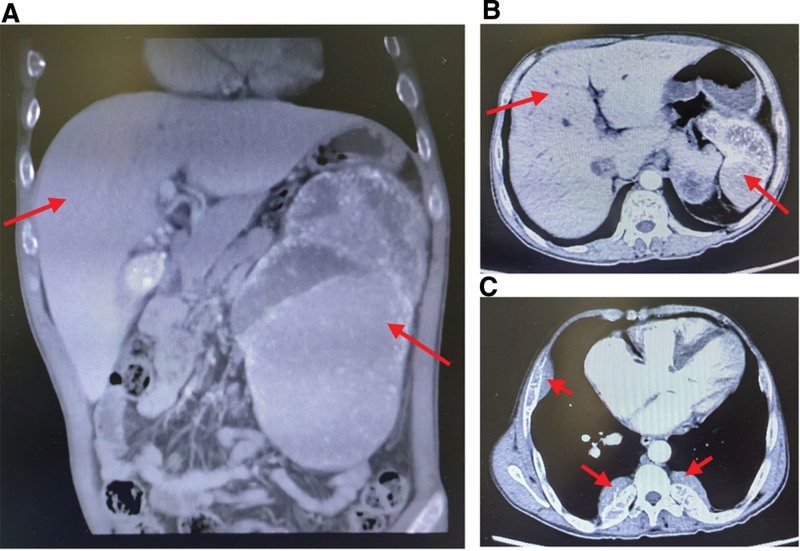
Follow-up computed tomography (CT) images 2 years after the first visit showing (A, B) hepatosplenomegaly and (C) extramedullary hematopoiesis in the ribs.
